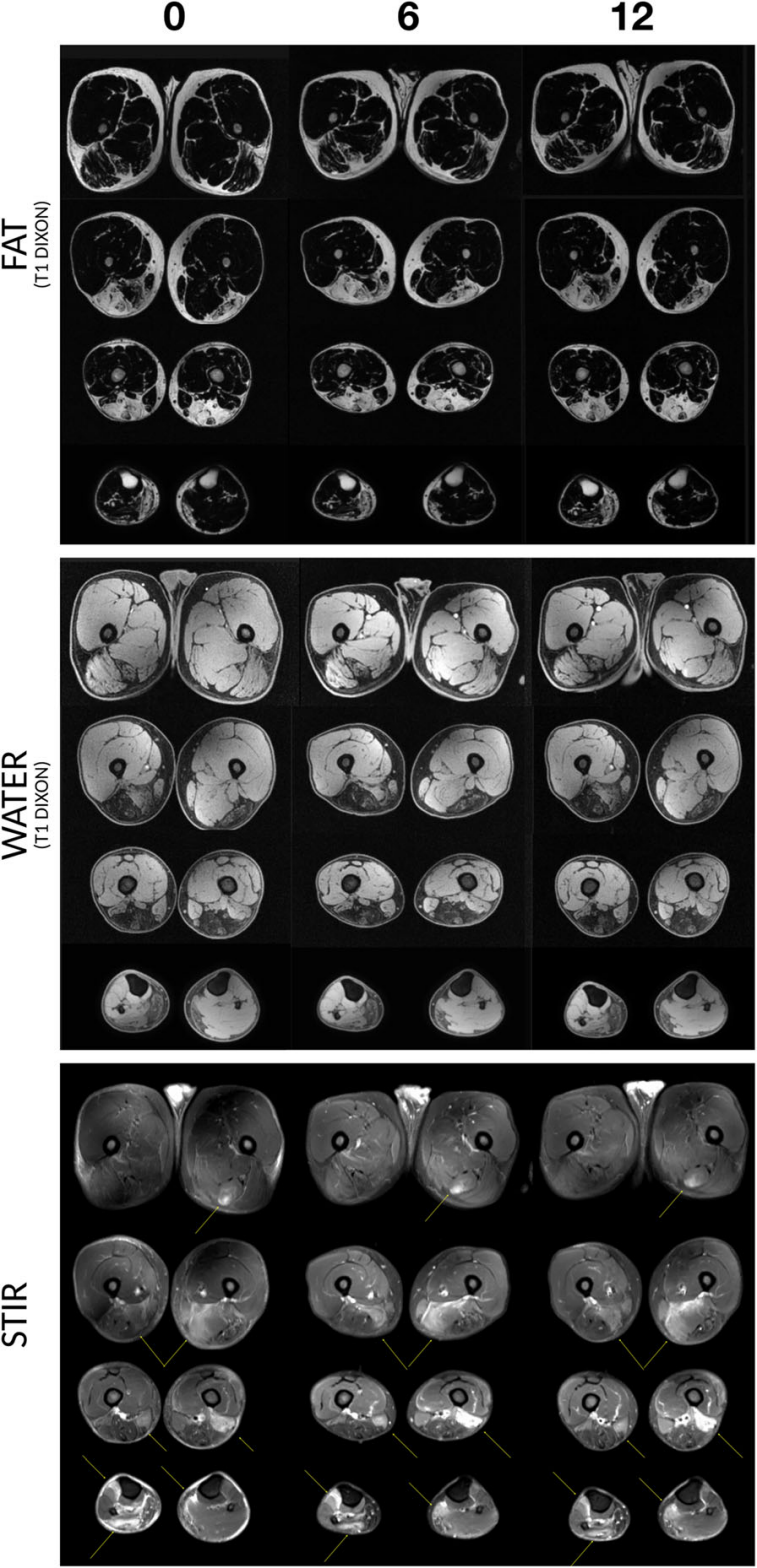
STIR and T1 MRI of lower extremity over the treatment period (6 and 12 weeks). The fat and water images derived from the T1 DIXON scan are shown at top and middle throughout the leg volumes across the study interval. In the bottom panel, regions with STIR+ signal are shown with arrows. The STIR+ signal persists at the two treatment time-points
